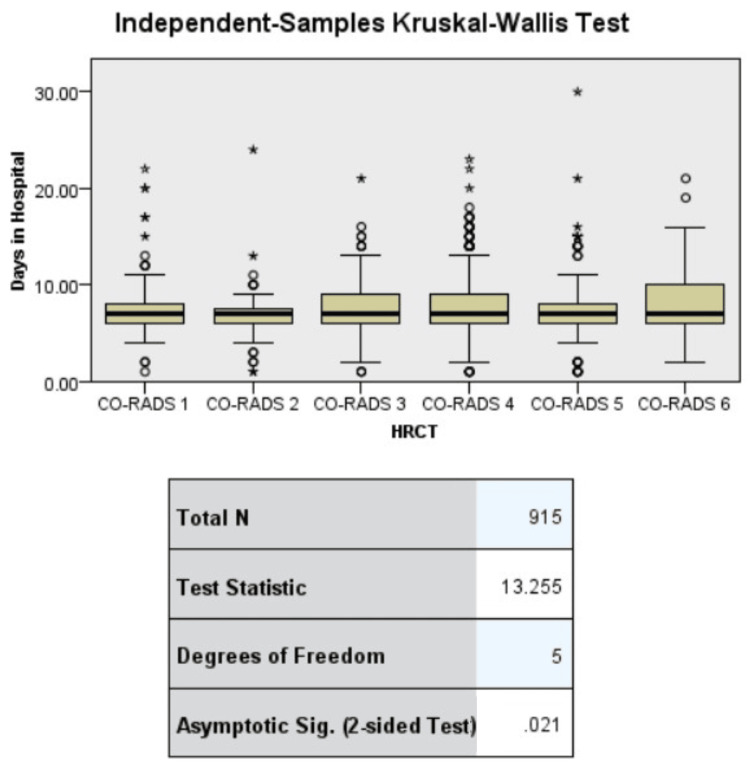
Association of high-resolution computed tomography with (a) symptomatic days and (b) days in hospital. HRCT: high-resolution computed tomography; CO-RADS: coronavirus disease 2019 reporting and data system
